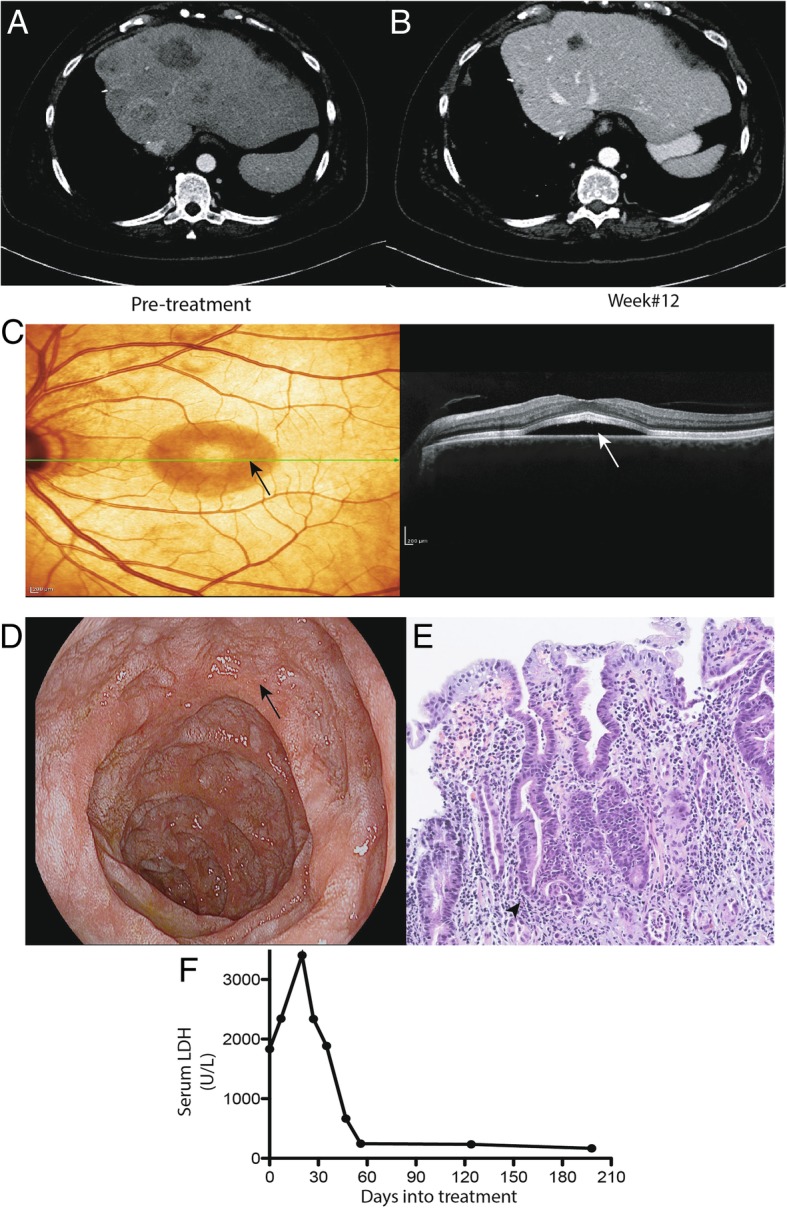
Clinical Characteristics. Panel a and b depict pre- and post-treatment computed tomography of the liver with complete resolution of liver metastases. Panel c depicts central serous retinopathy (arrow) on fundoscopic examination and Optical Coherence Tomography (OCT). Panel d and e shows endoscopic and pathologic findings of post-treatment duodenitis (arrow) with marked acute inflammatory cell infiltrate involving most of the glandular epithelium. The infiltrate is predominantly within deep crypt spaces (arrowhead). Panel f shows decline of serum LDH shortly after immunotherapy were initiated
